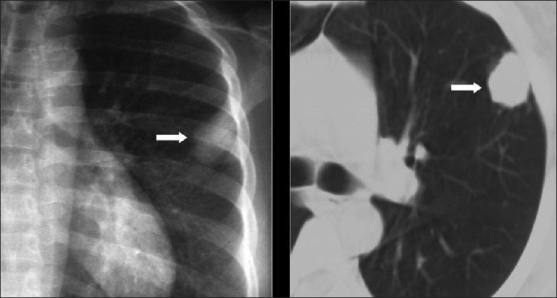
Cryptococcosis. Chest X-ray and computed tomography show a solitary pulmonary nodule. The diagnosis of Cryptococcosis was confirmed on biopsy
